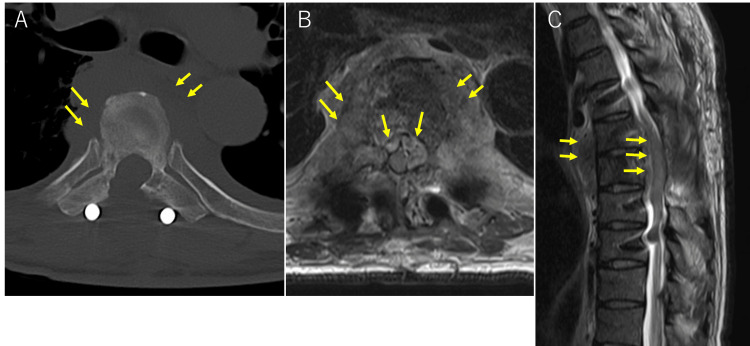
Postoperative CT and MRI after two weeks from the first surgery Axial plane CT (A), axial (B), and sagittal (C) T2-weighted MRI revealed a soft-tissue mass, which extended to the epidural space and paravertebral lesion.
